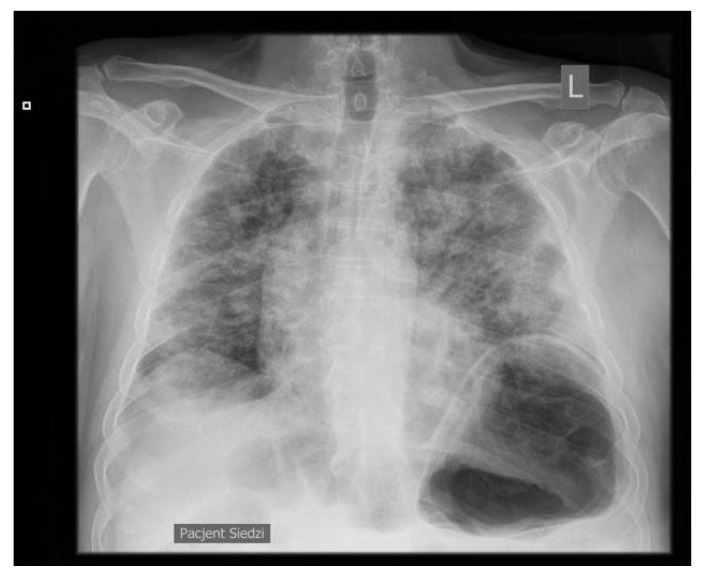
Chest X-ray. L—left side.
